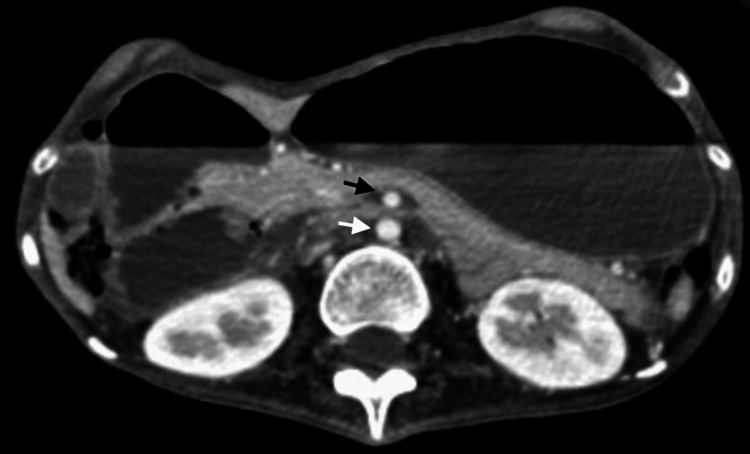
Abdominopelvic CT scan (axial view) revealing a distended stomach and the first part of the duodenum, with a narrowed aortomesenteric distance (8 mm), which is indicative of Wilkie syndrome CT: computed tomography The white arrow shows the abdominal aorta; the black arrow shows the superior mesenteric artery
